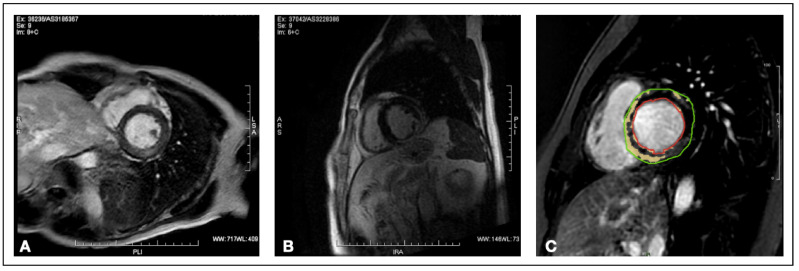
T1-weighted cardiac magnetic resonance short-axis of patients with different degrees of delayed enhancement. (A) Patient with mild presence of delayed gadolinium enhancement. (B) Patient with diffuse, sub-epicardial and mid-wall localization of delayed gadolinium enhancement. (C) Late gadolinium enhancement mass quantification technique. The green circle represents the sub-epicardial layers of myocardium, the red circle represents the sub-endocardial layers.
